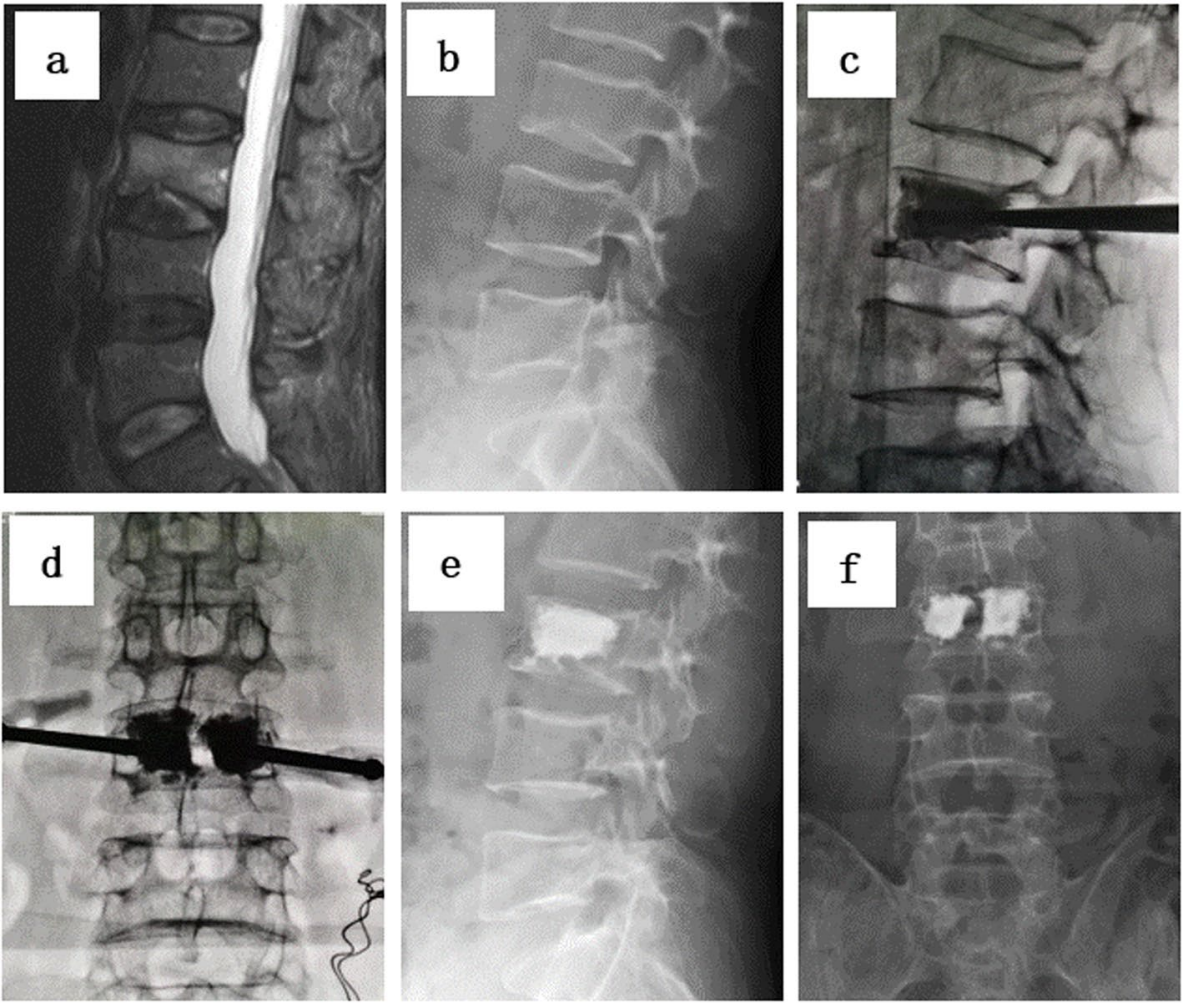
a-f Percutaneous kyphoplasty for the treatment of a L3 vertebral compression fracture in bipedicular approach. a-b Preoperative lateral radiograph and MRI showing a L3 vertebral compression fracture. c-d intraoperative view (e-f) Postoperative lateral radiograph showing cement distribution after undergoing PKP surgery of L3 vertebral compression fracture
